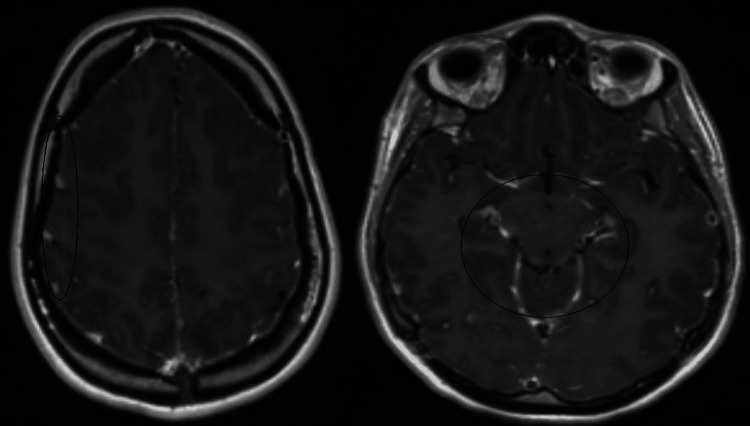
Postgadolinium contrast T1-weighted MRI head axial section at the level near the vertex (left) and postgadolinium contrast T1-weighted MRI head axial section at the level of the midbrain (right). Prominent enhancement within the sulcal spaces of both cerebral hemispheres, likely vascular in nature, although leptomeningeal enhancement cannot be entirely excluded. No definite cranial nerve enhancement
